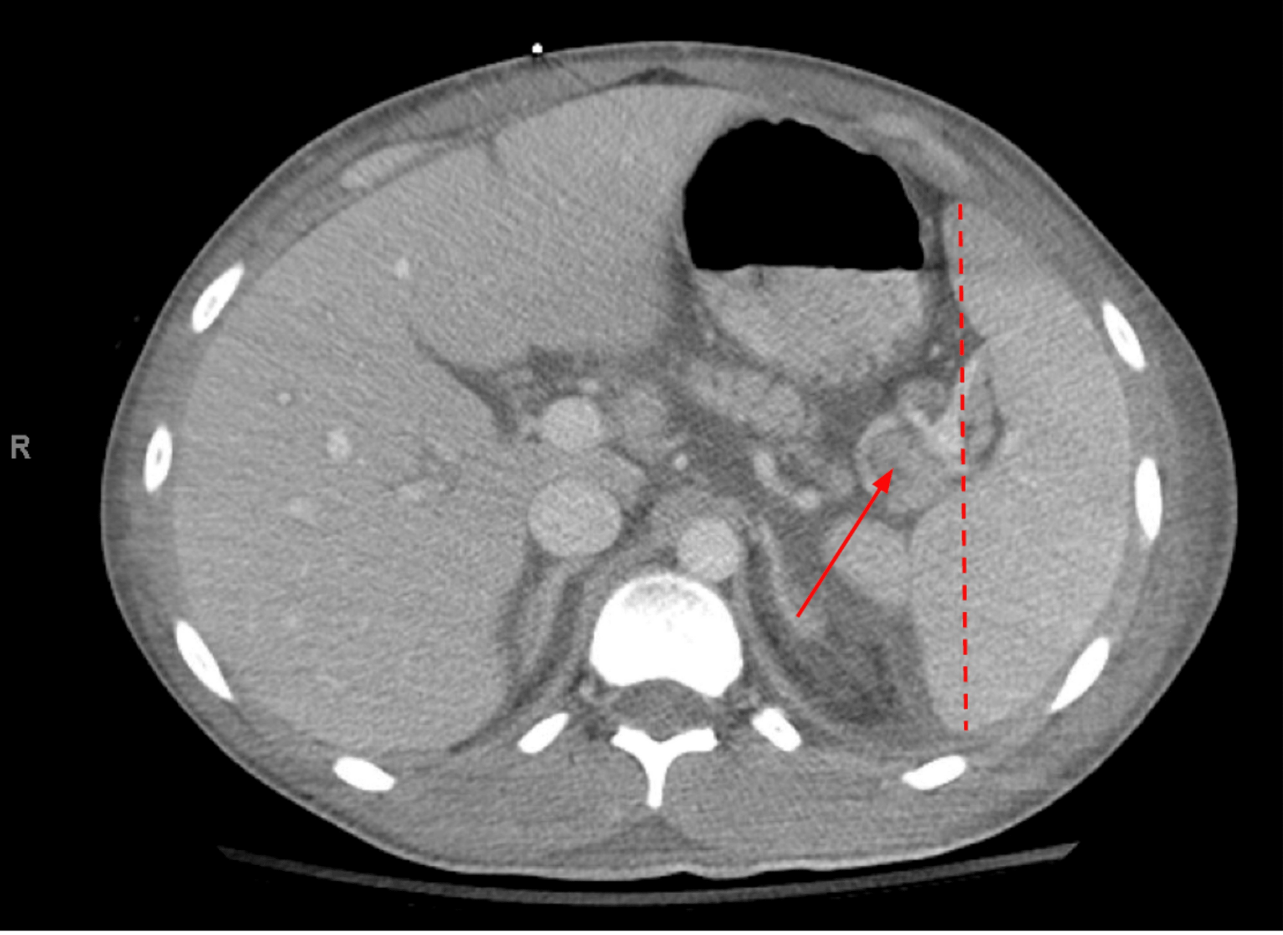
Computed tomography of the chest, abdomen, and pelvis: cross-section The red dashed line represents the length of the spleen, which is mildly enlarged at 14.3 centimeters (normal length <13 centimeters); the red arrow points to an enlarged lymph node within the splenic hilum, measuring near 10 millimeters, which is favored to be reactive.
